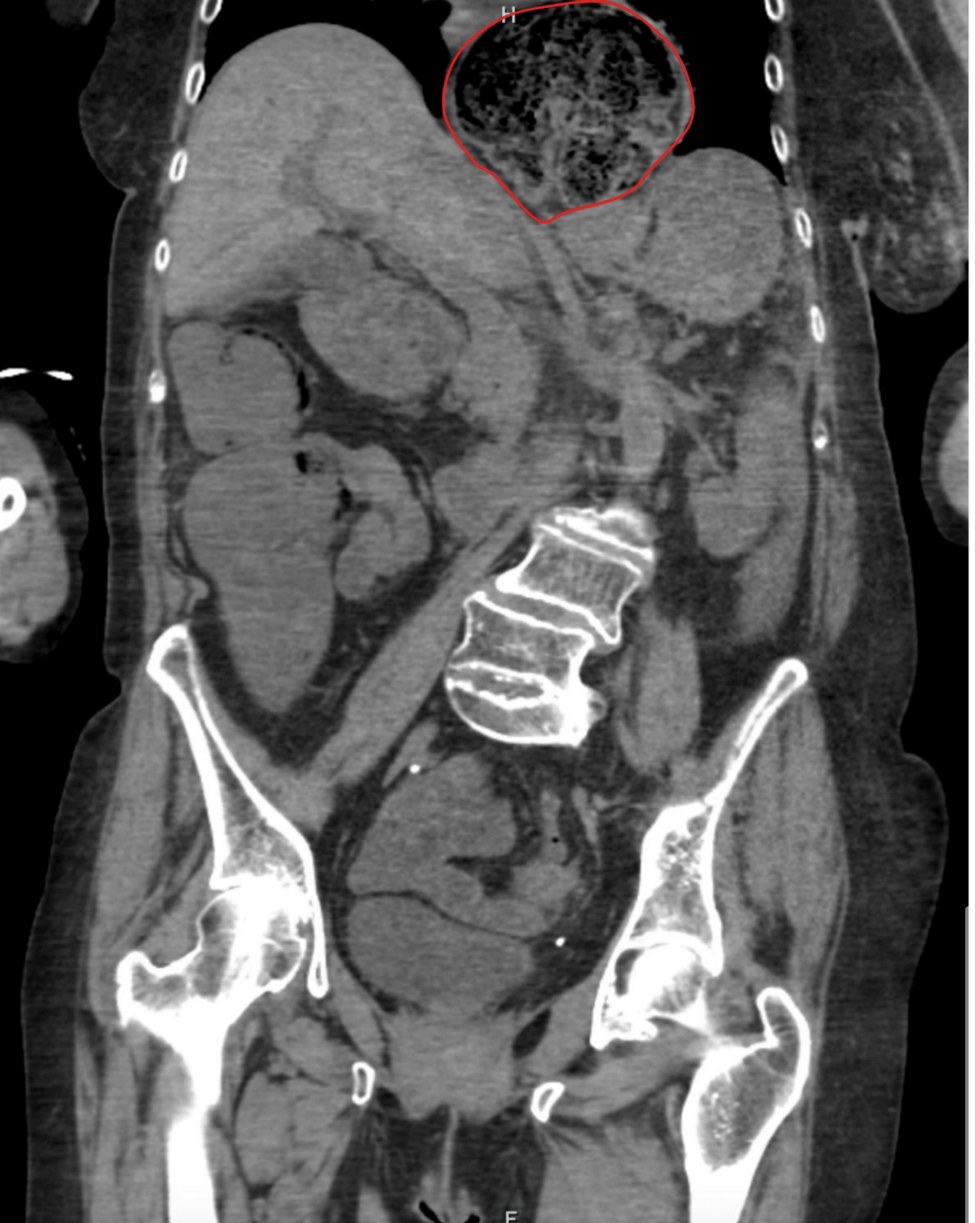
AP view of the CT abdomen and pelvis without contrast showing compression at the level of the aortic hiatus due to herniation of the apex of the sigmoid volvulus. The herniated bowel above the level of the diaphragm is delineated in red. AP: anterior-posterior; CT: computed tomography
